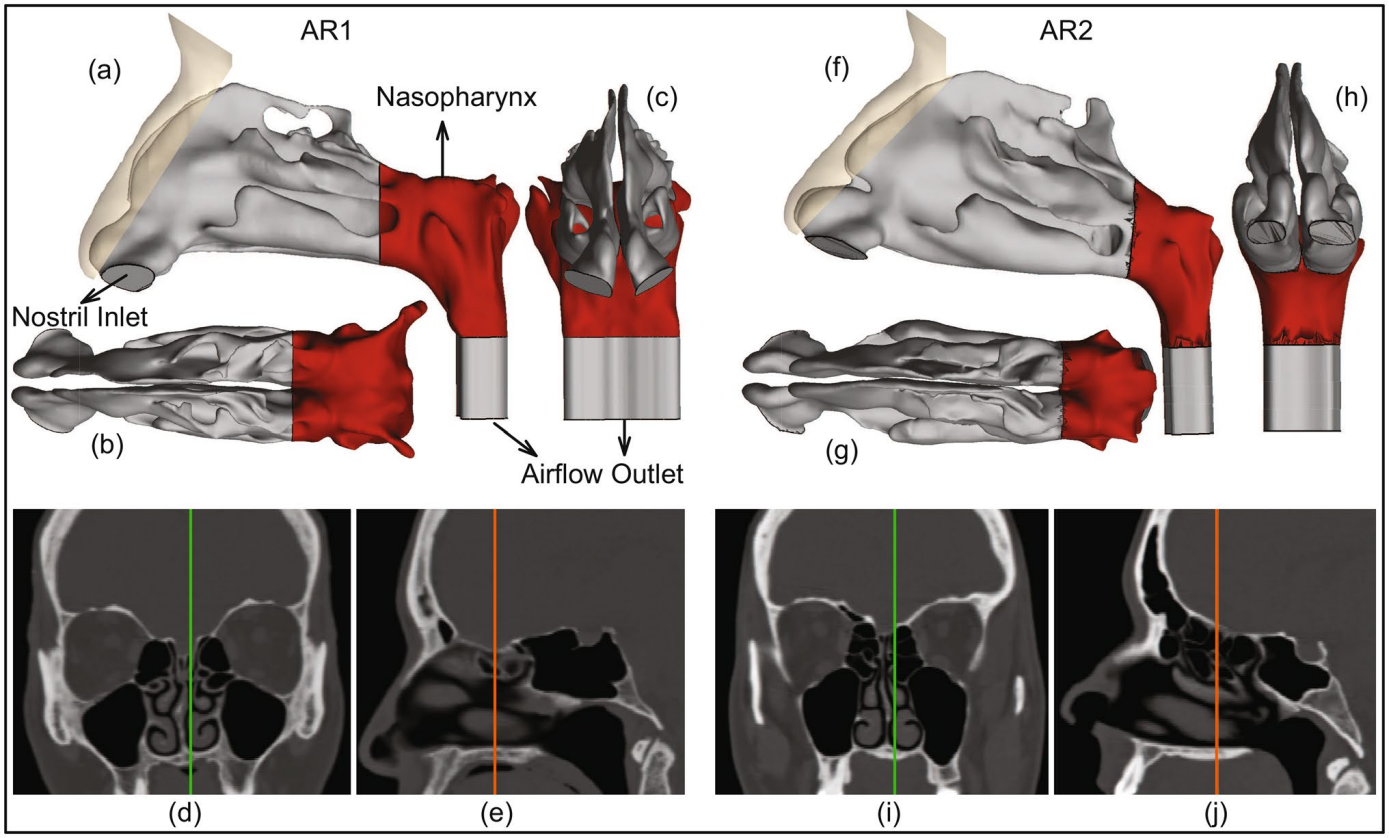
Anatomically realistic nasal airway geometries:  (a–c) respectively depict the sagittal, axial, and coronal views of the computed tomography (CT) based nasal domain in anatomic reconstruction 1 (AR1). (d,e) Representative CT slices for AR1. Therein, the green line in (d) corresponds to the location of the sagittal section shown in (e); the orange line in (e) corresponds to the location of the coronal section shown in (d). (f–h) Respectively depict the sagittal, axial, and coronal views of the CT-based nasal domain in anatomic reconstruction 2 (AR2). (i,j) Representative CT slices for AR2. Therein, the green line in (i) corresponds to the location of the sagittal section shown in (j); the orange line in (j) corresponds to the location of the coronal section shown in (i). The digital reconstructions only consider the main nasal cavity and leave out the sinus appendages. Nasopharynx is marked in red on the top row graphics. Visuals in (a–c), (f–h) are generated using the software ICEM CFD 15.0 (ANSYS Inc., Canonsburg, Pennsylvania; link to software homepage). The scans on (d,e) and (i,j) are extracted from the image processing software Mimics Research 18.0 (Materialise, Plymouth, Michigan; link to software homepage).
